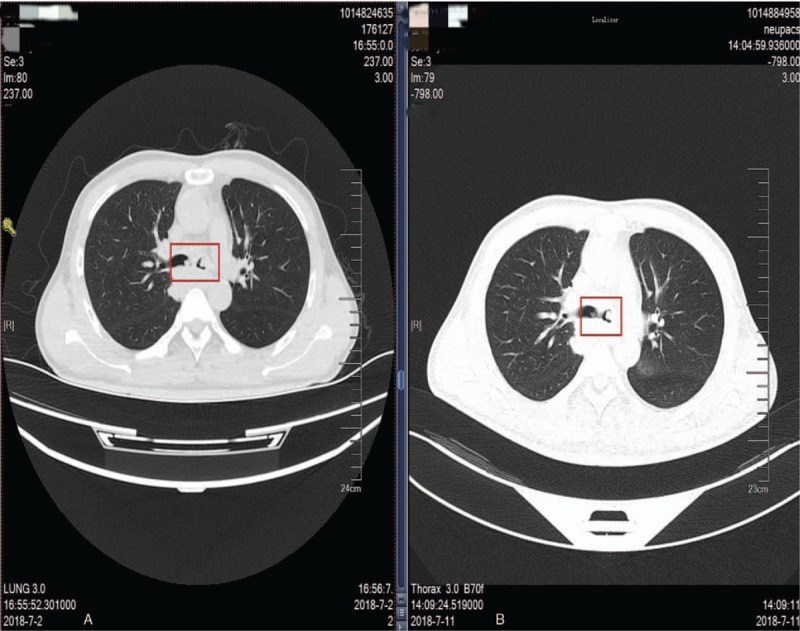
Chest CT at left main bronchus level of different periods. (A) Chest CT at left main bronchus level before RPE. (B) Chest CT at left main bronchus level after RPE. CT = computed tomography, RPE = re-expansion pulmonary edema.
